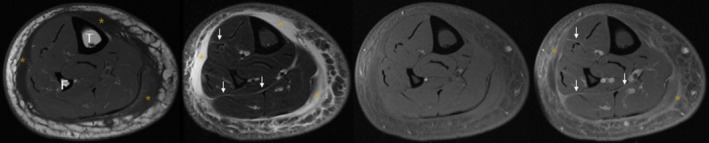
Initial magnetic resonance imaging of the right lower extremity (axial plane from left to right with TR/TE times [in msec]: T1 [564/12], T2 fat‐suppressed [4517/70], T1 precontrast fat‐suppressed [599/12], and T1 postcontrast fat‐suppressed [599/12]) demonstrates extensive circumferential edema and enhancement of the superficial soft tissues and superficial fascia (asterisks) and, to a lesser extent, the deep fascia (arrows)
